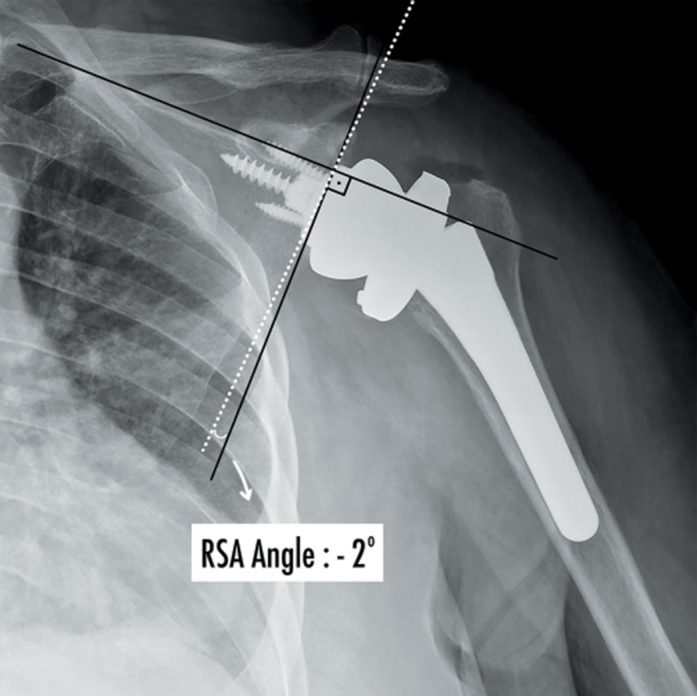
Postoperative X-ray demonstrate measurement of the RSA angle in lateralized onlay prosthesis. RSA, Reverse shoulder arthroplasty.
